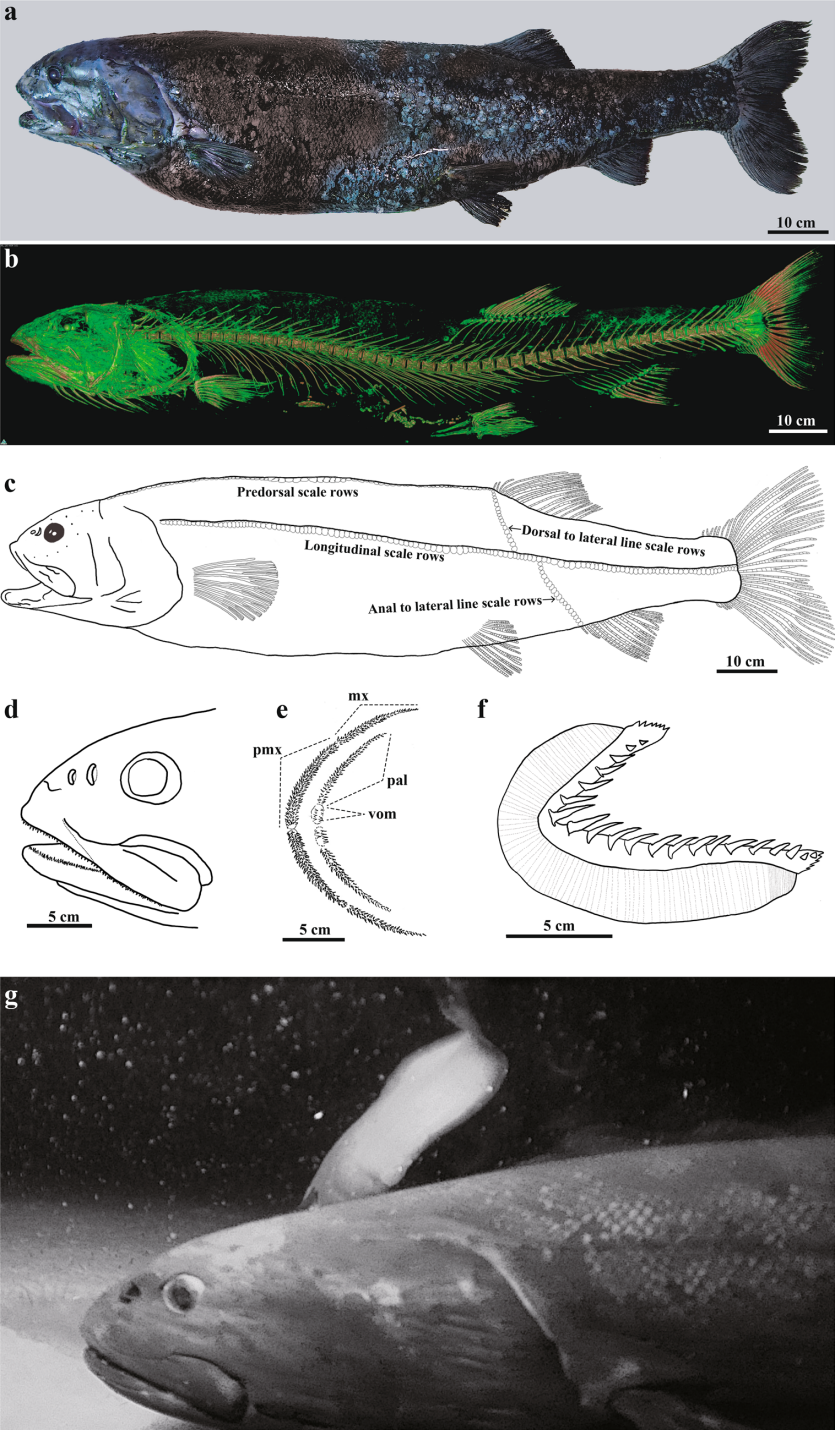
Narcetes shonanmaruae*. (a), (b), (d), (e) holotype (SH8-69). (c) paratype 3 (SH12-14). (f) paratype 2 (SH12-1). (a) Lateral view. (b) Computed tomography image of the endoskeleton. (c) Lateral view. Scale rows indicated. Lateral line scales not shown. (d) Lateral view of head. (e) Tooth pattern of upper jaw. mx: maxillary, pal: platine, pmx:premaxillary, vom: vomer. (f) Gill raker. Right side of first arch. (g) In situ video grab of active swimming individual recorded using a baited camera.
